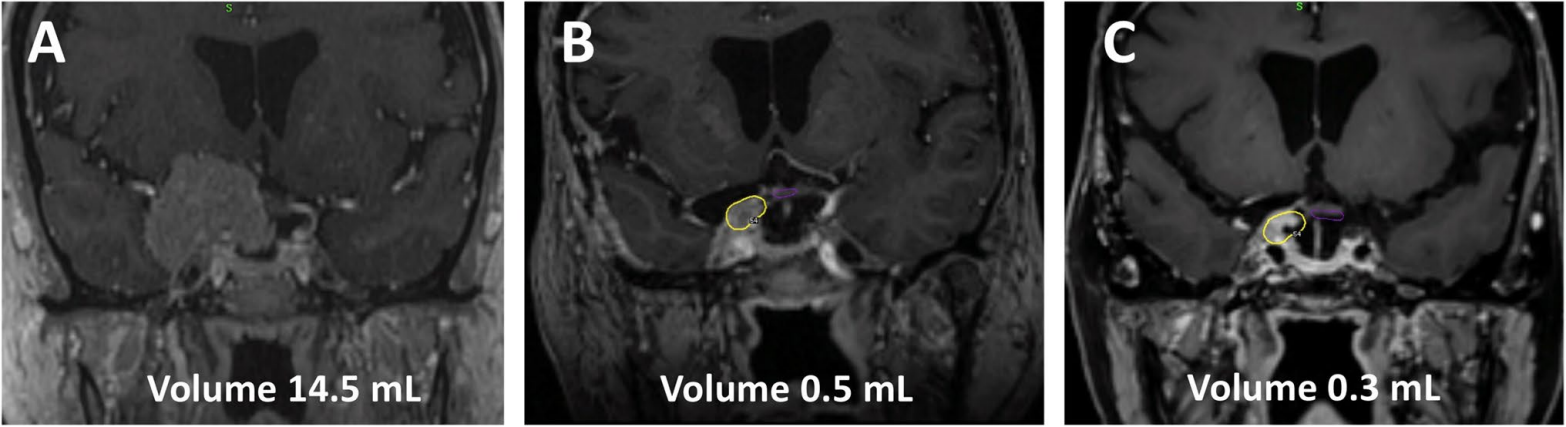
Gd-enhanced T1W coronal MR images showing the preoperative tumor (A) and residual volume at 3-month (B) and 1-year (C) follow-up
